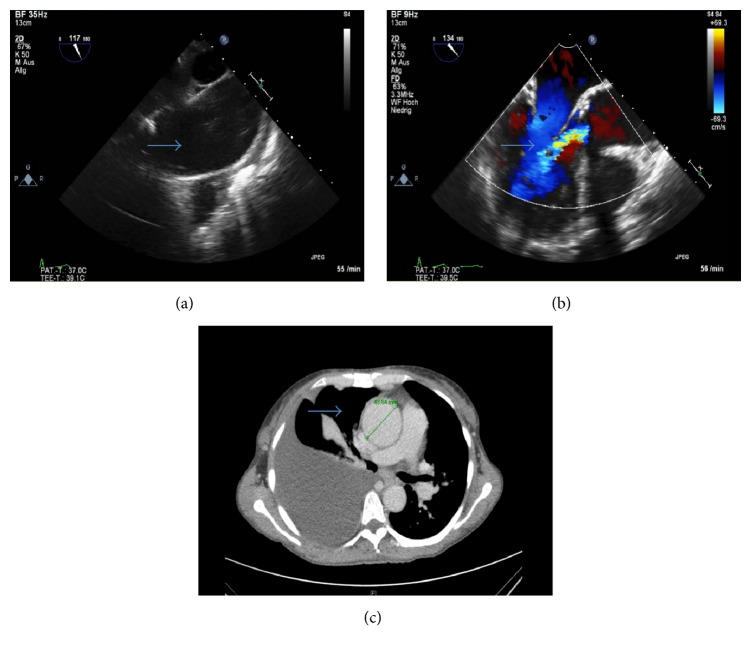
Aneurysm of ascending aorta ((a), blue arrow) and insufficiency of the aortic valve ((b), blue arrow, transesophageal echocardiography). Ascendens aneurysm was evaluated using CT-scan ((c), blue arrow, distal part of the aorta ascendens above the sinus valsalvae, early arterial phase).
